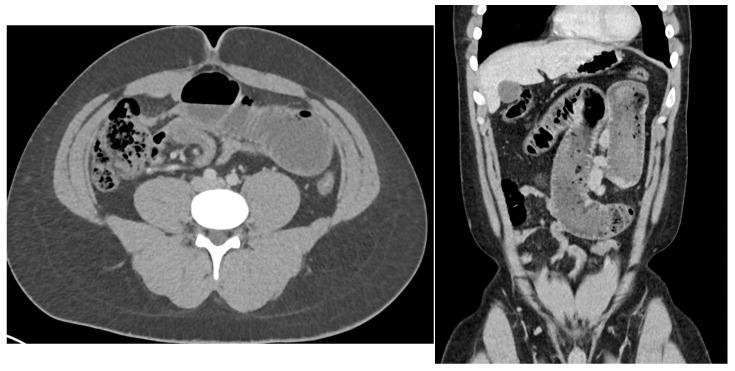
CT abdomen/pelvis in the axial and coronal views revealing a high-grade small bowel obstruction with mesenteric twisting.
